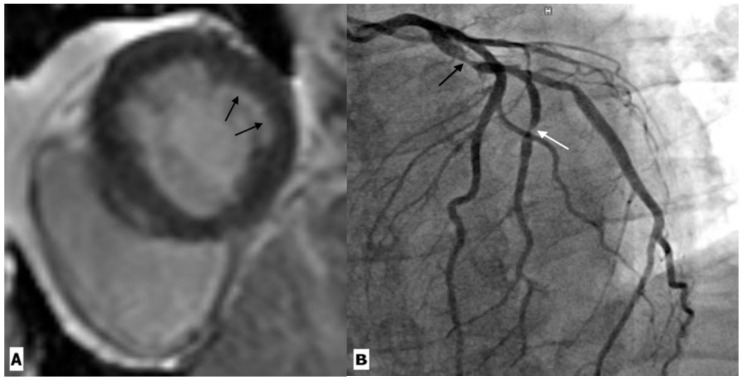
Cardiac magnetic resonance imaging in a 59-year-old HIV-infected male patient with coronary artery disease. (A) Basal ventricular short-axis view gadolinium enhancement image showing subendocardial enhancement in the inferolateral segment (black arrow). (B) Coronary angiography revealed severe stenosis in the proximal left circumflex artery (black arrow) and its first obtuse marginal branch (white arrow).
